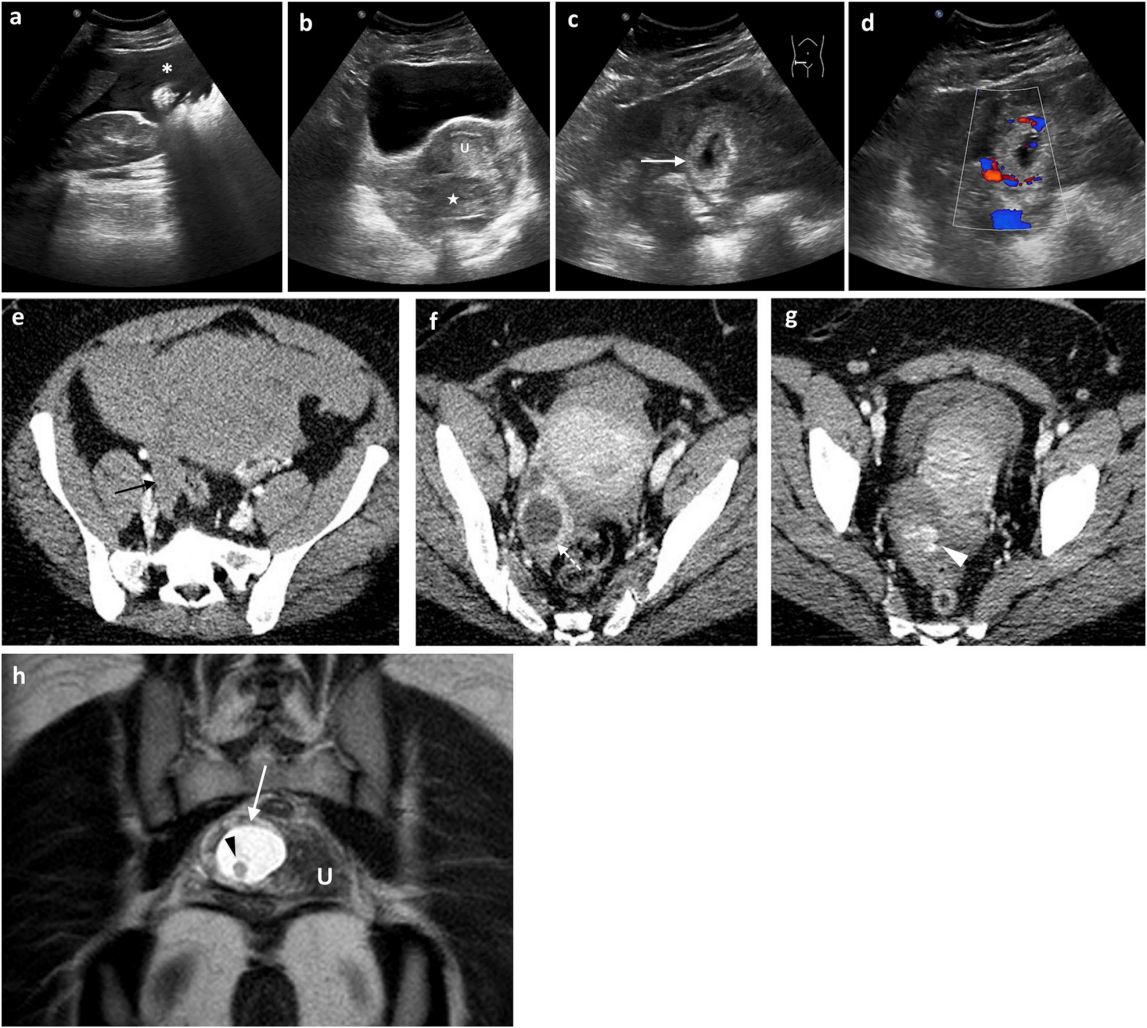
Ectopic pregnancies in three different patients. a–c Teenager with intense abdominal pain and persistent vaginal bleeding. Grey-scale US images show a large amount of partially echogenic abdominal fluid (asterisk) and a pelvic haematoma (star) surrounding the uterus (U). A round, thick-walled para-uterine cystic mass (arrow) with vascularised wall and a fetal pole was noted with Colour Doppler (d). No gestational sac was identified within the endometrial cavity. Laparoscopic surgery confirmed a ruptured ectopic tubal pregnancy with extensive hemoperitoneum. e–g Axial post-contrast CT images in a different patient with a ruptured tubal EP. Normal right adnexa is noted (black arrow), separate from a ring-enhancing right para-uterine cystic mass (dotted arrow) which increases the likelihood that the cystic mass represents an ectopic pregnancy. Active bleeding from the ruptured tubal pregnancy is visible during the arterial phase (arrowhead). h Woman in her 20’s presenting in the emergency department with acute pelvic pain and vaginal bleeding. Coronal T2 WI shows an eccentric gestational sac (arrow) with a fetal pole (arrowhead) located in the interstitial segment of the right Fallopian tube, next to the uterus (U). MRI is more helpful in evaluating ectopic interstitial pregnancy, which is a diagnostic challenge on ultrasound
